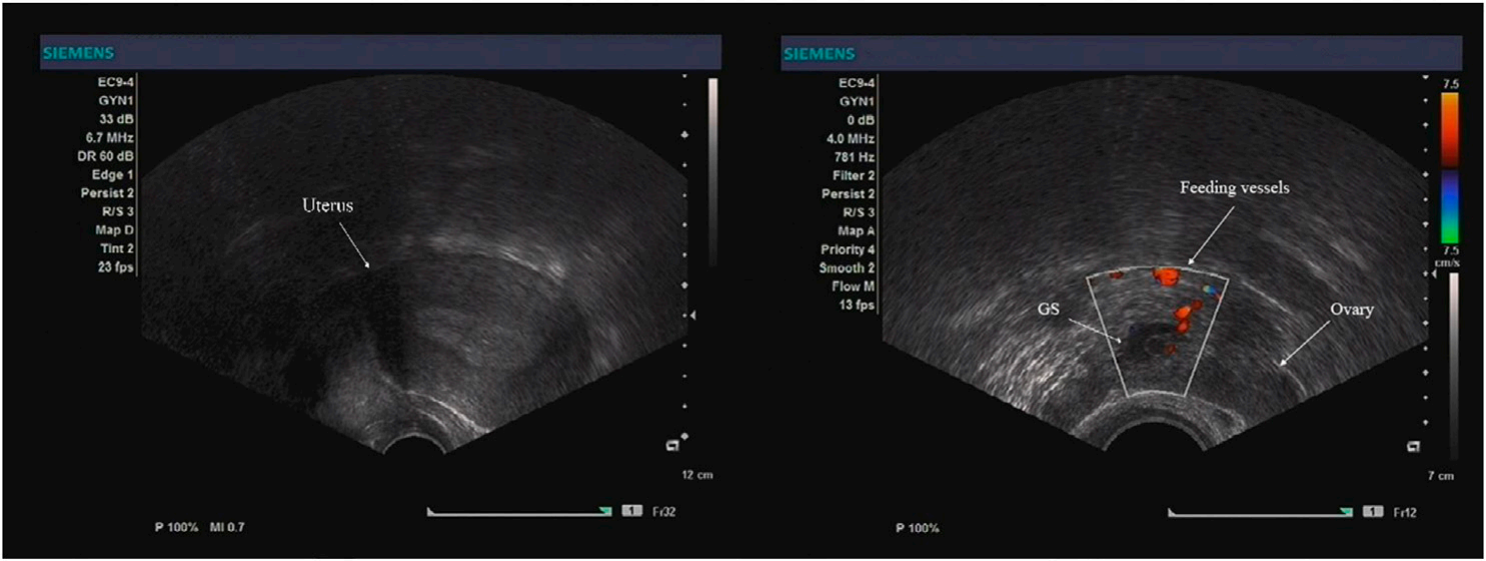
Ultrasound image of the left ovarian ectopic pregnancy, showing the GS with a yolk sac inside and feeding vessels around.
